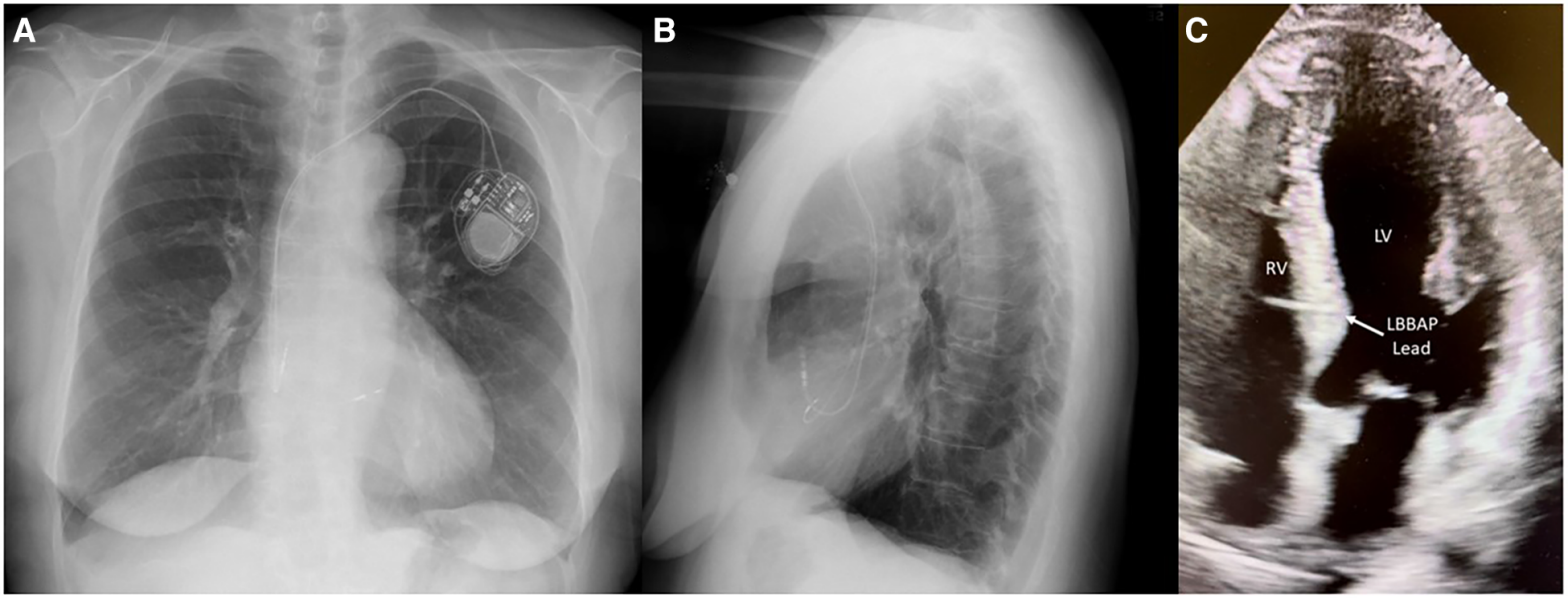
Medtronic 3830 lead with a fixed helix implanted through the RV endocardium to the LV endocardium in the mid-interventricular septum capturing the left bundle branch of the conduction system. (A) PA chest x-ray, (B) lateral chest x-ray, and (C) four-chamber transthoracic echocardiogram view of the left bundle branch pacing lead.
